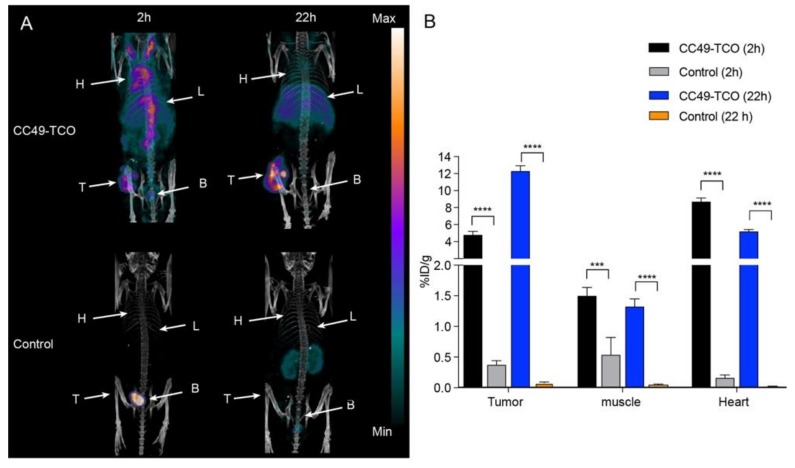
Pretargeted SPECT/CT imaging of [^111^In]2 targeting CC49-TCO (5), which was administered 24 h prior to the administration of [^111^In]2. (A) Respective SPECT/CT images after 2 h and 22 h. (B) Image-derived quantitative analysis of tissue uptake. * *p* < 0.001 and  *p* < 0.0001. Each image is scaled between its minimum and maximum pixel intensity.
